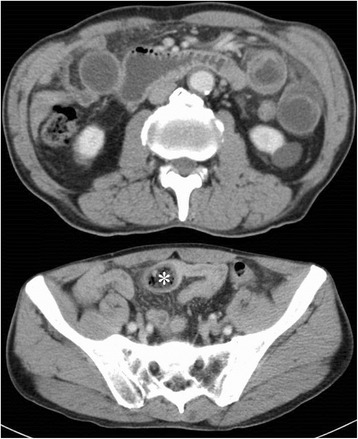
Computed tomography performed the day after esophagogastroduodenoscopy. The bezoar had disappeared from the duodenum and moved through the ileum (*)
